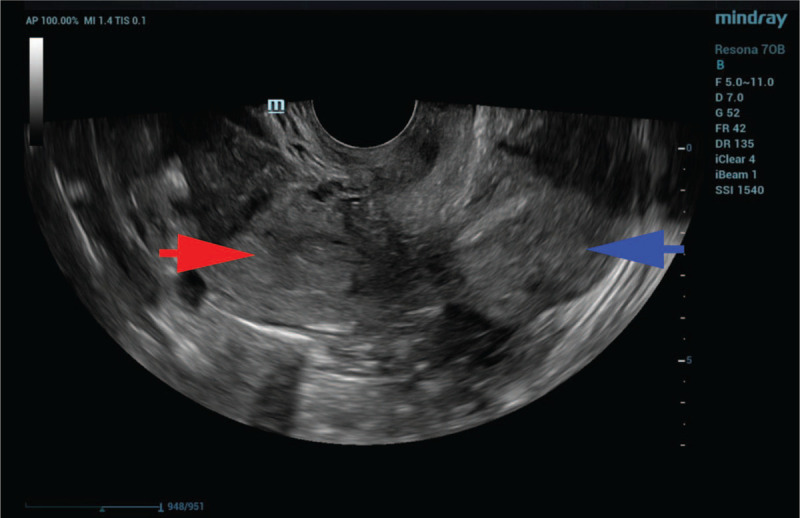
Transvaginal ultrasound before surgery confirmed a didelphic uterus. The red arrow indicates the right uterus. The blue arrow indicates the left uterus.
